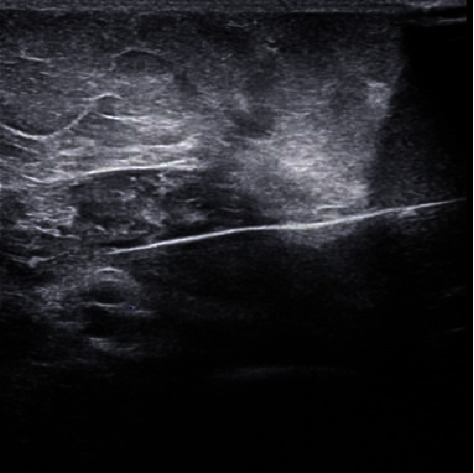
Transverse sonographic view of the median nerve proximal to the left antecubital fossa, with an in-plane stimulator needle superior to the median nerve. Due to poor quality, the sonographic images of the other two cases were not included.
